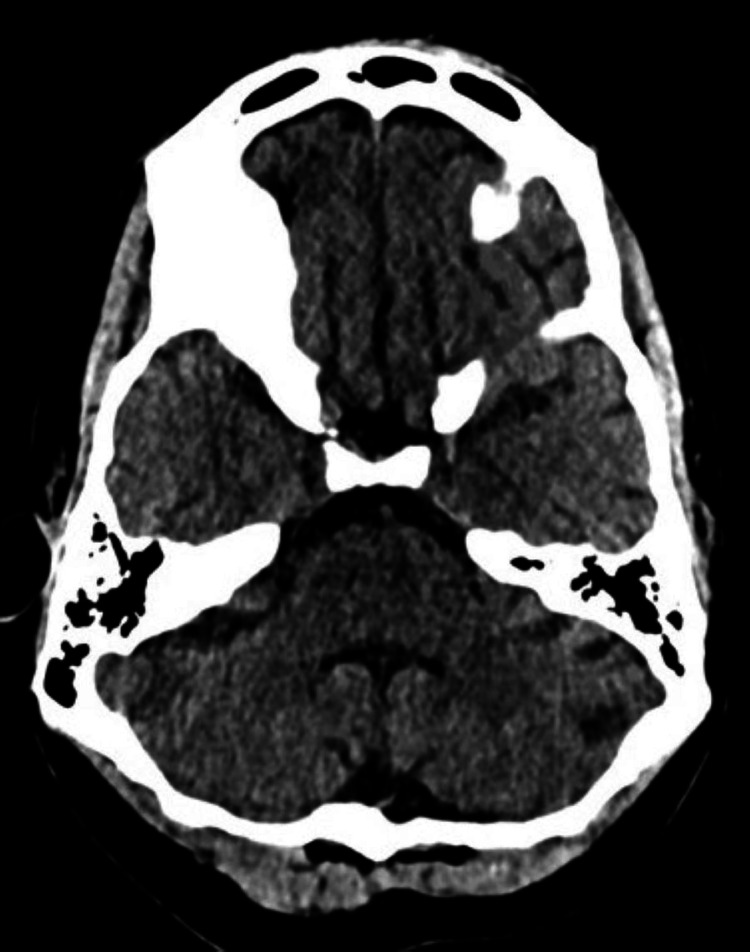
Axial plain CT head at initial admission was reported as nil acute with no evidence of any abnormality
